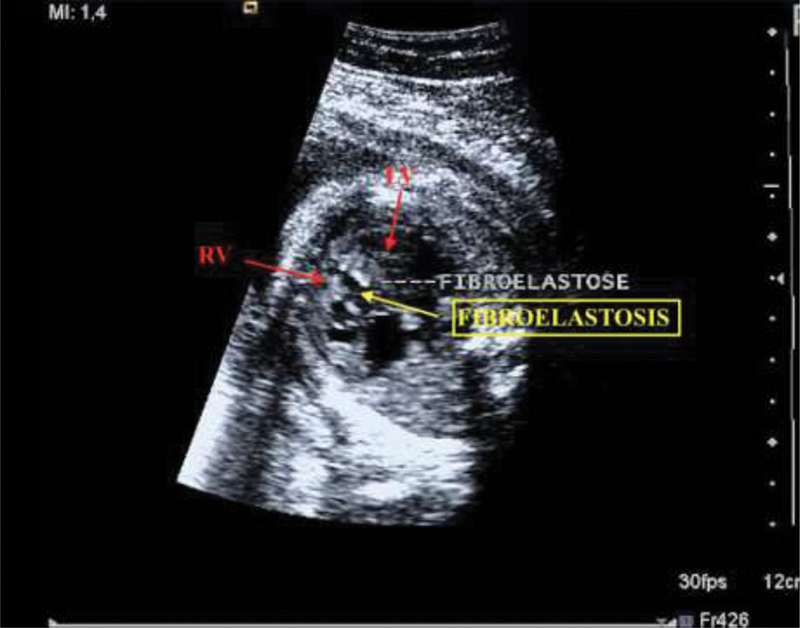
Fetal echocardiogram performed at 32 weeks of gestation showing signs of RV fibroelastosis. Abbreviations: RV: right ventricle, LV: left ventricle; HR: heart rate.
